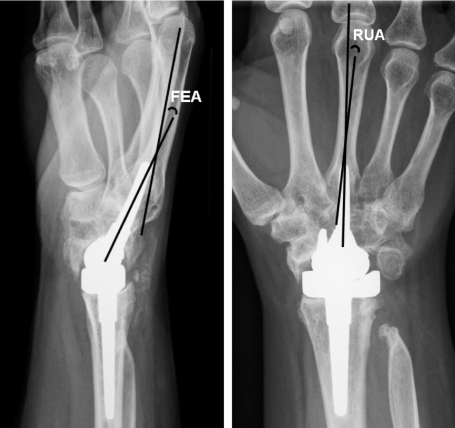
Left panel: The flexion-extension angle (FEA) is the angle between the line through the middle of the metacarpal stem and the middle of the third metacarpal on the lateral radiograph. Right panel: Radial-ulnar angle (RUA) is the angle between the middle of the metacarpal stem and the middle of the third metacarpal on the anterior-posterior radiograph.
